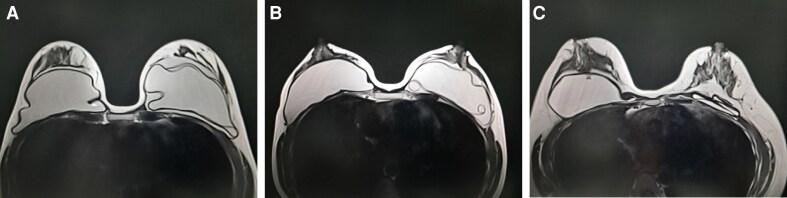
(A) MRI findings of intracapsular rupture showing linguine sign. (B) MRI findings of intracapsular rupture showing teardrop sign. (C) MRI findings showing extracapsular rupture.
